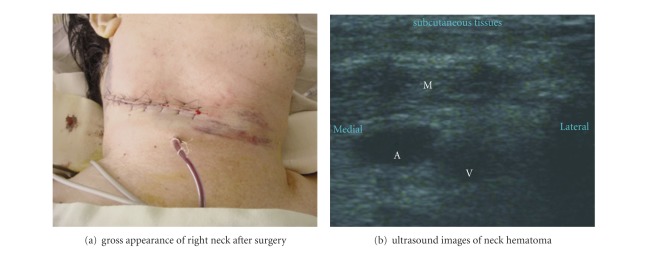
Delayed right neck hematoma. (a) Gross appearance of the neck after surgery. (b) Ultrasound image of right neck showed edematous muscles and swelling connective tissues. No thrombus in the jugular vein: common carotid artery (A), internal jugular vein (V), and edematous muscle layers (M).
